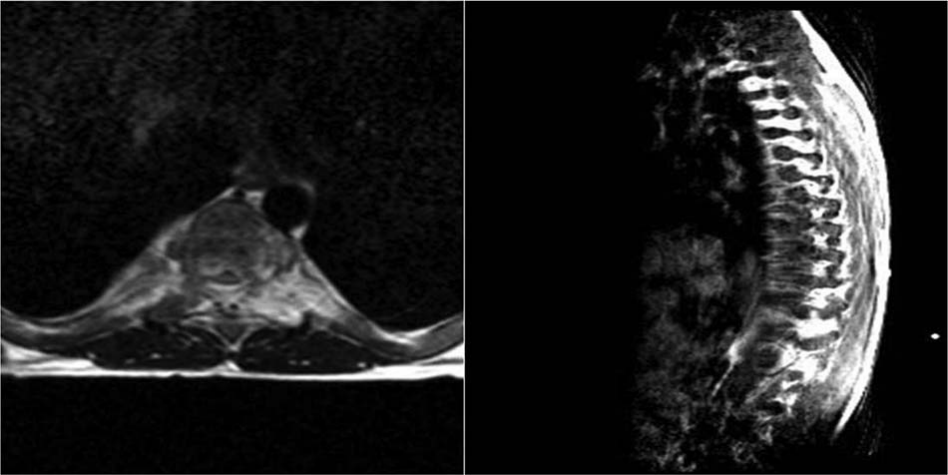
(A) Axial and (B) Para-sagittal T2W MRI of dorsal spine after radiation treatment reveals no evidence of lesion.
